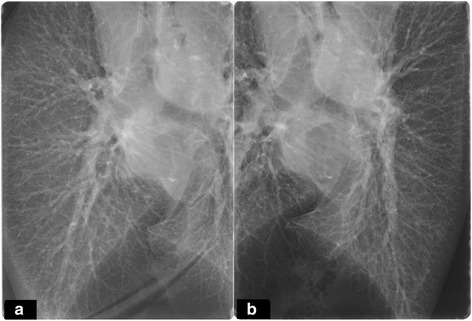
Lung X-ray in the OCS device showing no sign of oedema, infection or other abnormalities in the right (a) and in the left lung (b)
